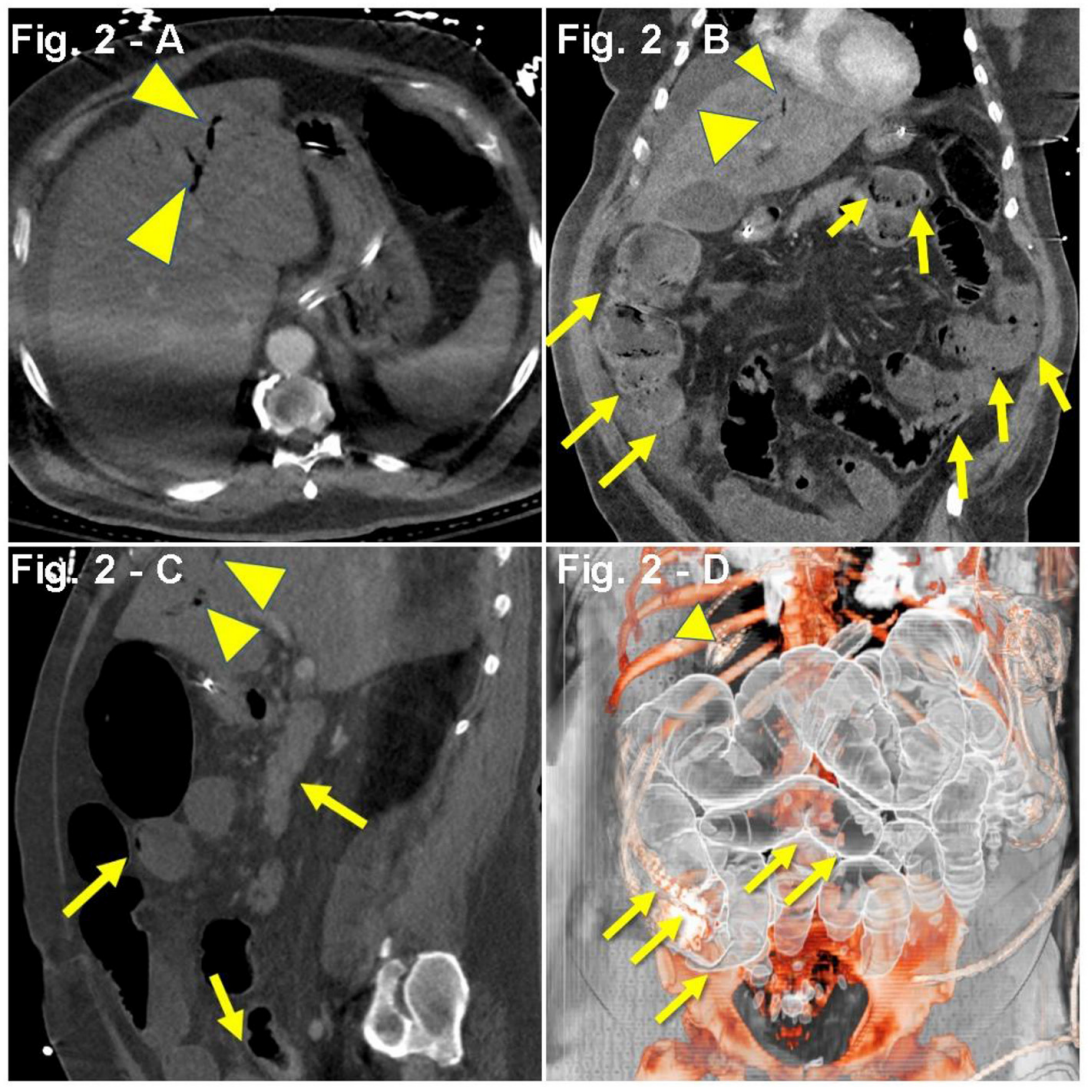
(A–D) Case presentation of a 61-year-old male COVID-19 patient with respiratory failure on TCZ with rising lactate, abdominal ileus, abdominal CT with intravenous and oral contrast, (A) axial, (B) coronal, (C) sagittal, and (D) 3D reconstruction, with ileus and small and large bowel dilatation, with small bowel and cecal pneumatosis (yellow arrows) with portal gas (yellow arrowheads), and splenic and mesenteric vein gas.
